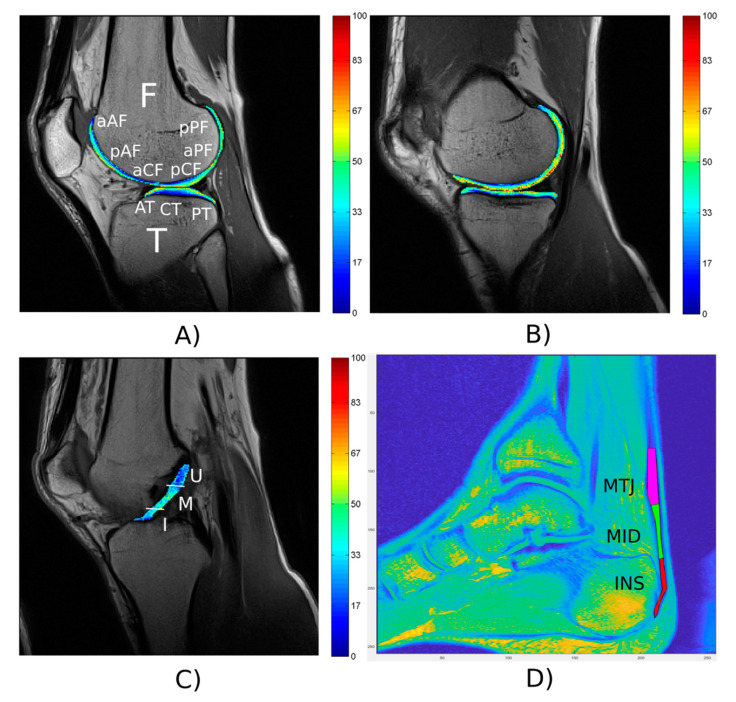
Representative T2 relaxation time map of knee articular cartilage and regions-of-interest (ROIs) for the lateral (A) and medial compartment (B); F—femur, aAF—anterior side of anterior part of femur, pAF—posterior side of anterior part of femur, aCF—anterior side of central part of femur, pCF—posterior side of central part of femur, aPF—anterior side of posterior part of femur, pPF—posterior side of posterior part of femur, T—tibia, AT—anterior part of tibia, CT—central part of tibia, PT—posterior part of tibia. Representative T2 relaxation time map and ROIs of ACL (C); Whole ACL, I—insertion, M—mid part, U—upper part). ROIs of AT on sagittal T2*-weighted image (D); INS—insertion, MID—middle, MTJ—muscle-tendon junction.
